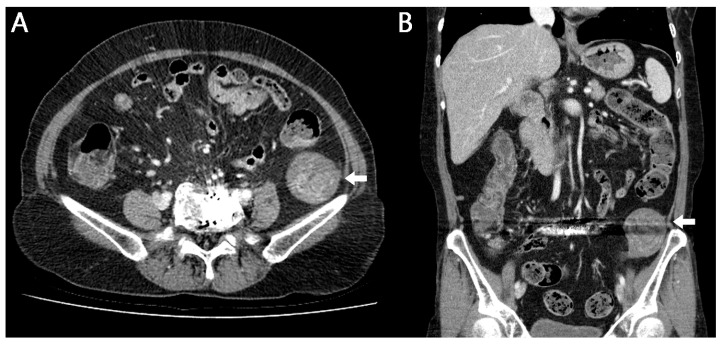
Retention of gauze, sponges, or various surgical instruments after surgery is rare [1]. Although this complication is entirely preventable, when it does occur, it may lead to severe, potentially life-threatening consequences. Nevertheless, the true incidence is likely underreported because of medicolegal concerns [2]. Gossypiboma, a term combining gossypium (Latin for cotton) and boma (Swahili for “to hide”), refers specifically to retained surgical textiles [3]. Gossypiboma is an uncommon yet significant postoperative complication resulting from retained surgical materials. Despite its rarity, it can present with a wide range of clinical manifestations and may lead to substantial morbidity. Its incidence, clinical features, and preventive strategies have been extensively discussed in the literature. Here, we report a case of gossypiboma discovered on abdominal computed tomography (CT) in a patient who presented to our hospital with abdominal pain. The diagnosis was confirmed following surgical resection of the abdominal mass. A 61-year-old female patient visited our hospital complaining of pain in the left lower abdomen that began 3 days ago. The patient said that she had a history of lumbar disc surgery at another hospital 10 years ago and the surgery was performed on the lower left abdomen. The patient had undergone lumbar disc surgery at another hospital, and although the exact surgical approach was not documented, the procedure was presumed to have been performed via a retroperitoneal approach based on imaging findings and the operative scar location. In addition, there was a history of coil embolization for subarachnoid hemorrhage 4 years ago. The physical examination revealed blood pressure 120/70 mmHg, pulse rate 68/min, respiration rate 20/min, and body temperature 36.5 °C and the patient showed acute illness. On abdominal examination, a relatively immobile mass without tenderness was palpable in the left pelvic region. Peripheral blood test showed white blood cell 6390/mm^3^ (Neutrophil 60.9%), hemoglobin 12.3 g/dL, and platelet 205,000/mm^3^. Biochemistry analysis showed aspartate aminotransferase (AST)/alanine aminotransferase (ALT) 17/12 IU/L, total/direct bilirubin 0.5/0.2 mg/dL, blood urea nitrogen 18 mg/dL, creatinine 0.5 mg/dL, and C-reactive protein (CRP) 0.5 mg/dL. Abdominal CT image: (A) axial and (B) coronal CT images demonstrate metal artifacts from previous lumbar disc surgery and an ovoid 4.5 × 4.7 × 6.1 cm sized poorly enhancing low-attenuation lesion with inner whirled high-attenuation portions in retroperitoneal space of left upper pelvic cavity (arrow).
